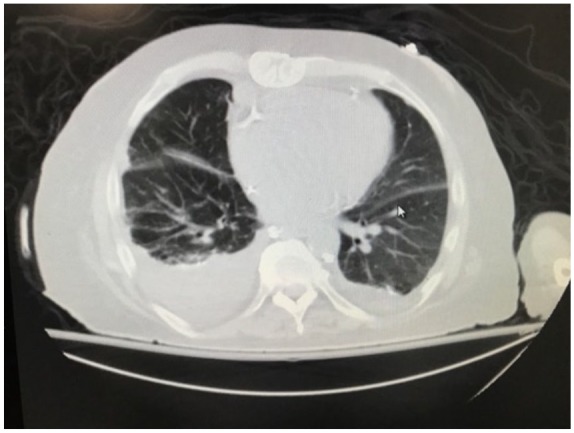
Computed tomography scan of chest showed findings consistent with right-sided
